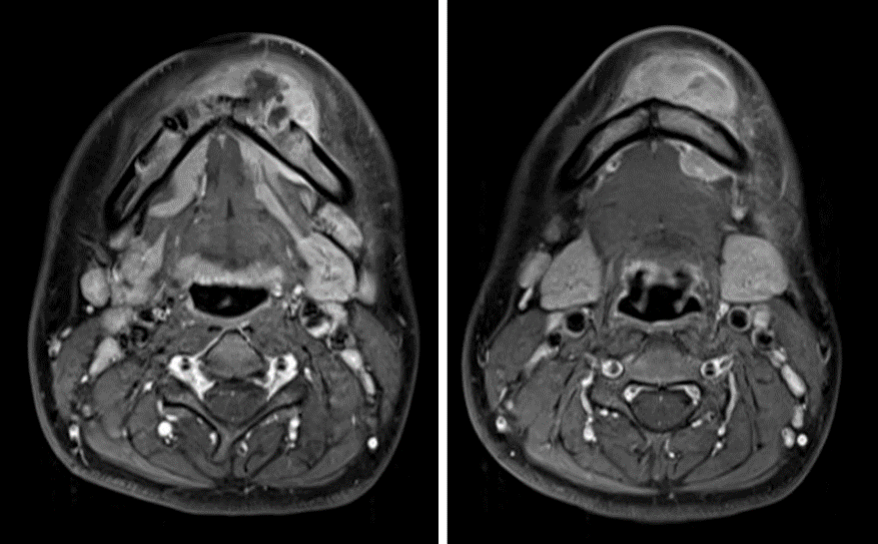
Transverse T1-weighted post contrast MR images reveal a destructive mandible lesion with extension to the adjacent soft tissue with inhomogeneous enhancement
